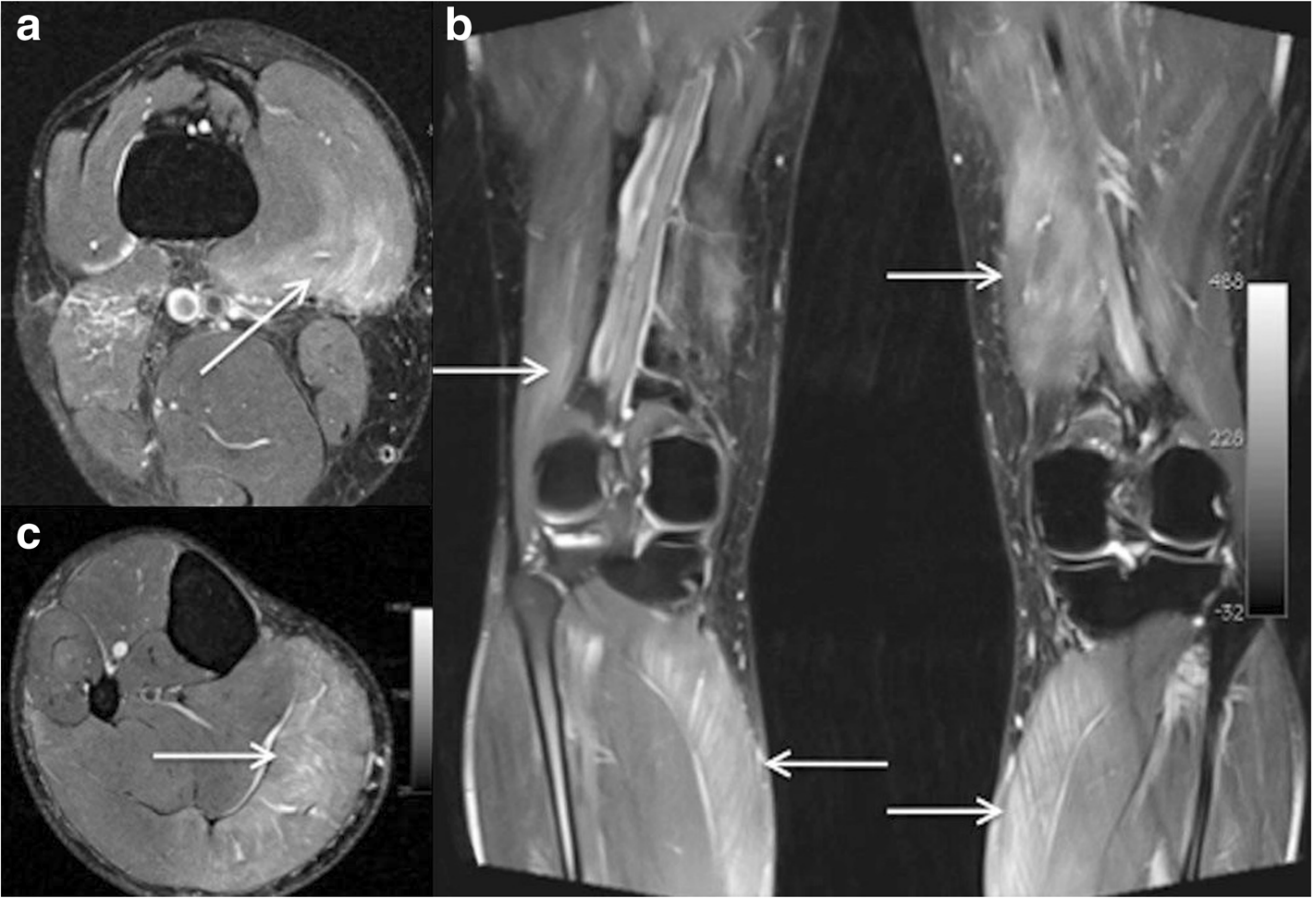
Axial (a, c) and coronal (b) PDf-weighted MR images in an athlete with delayed onset muscle soreness 4 days after completing a triathlon. There is an extensive lower limb edema bilaterally, predominately involving the vastus medialis and the medial head of gastrocnemius (arrows) (images with courtesy of Dr. Andrew Van Den Heever, Cape Town, South Africa). *Reprinted by permission from Springer: Springer Nature, Magnetic resonance imaging of the skeletal musculature, MRI of muscle injuries, Simon Dimmick, Christoph Rehnitz, Marc-André Weber, and James M. Linklater, 2014*
